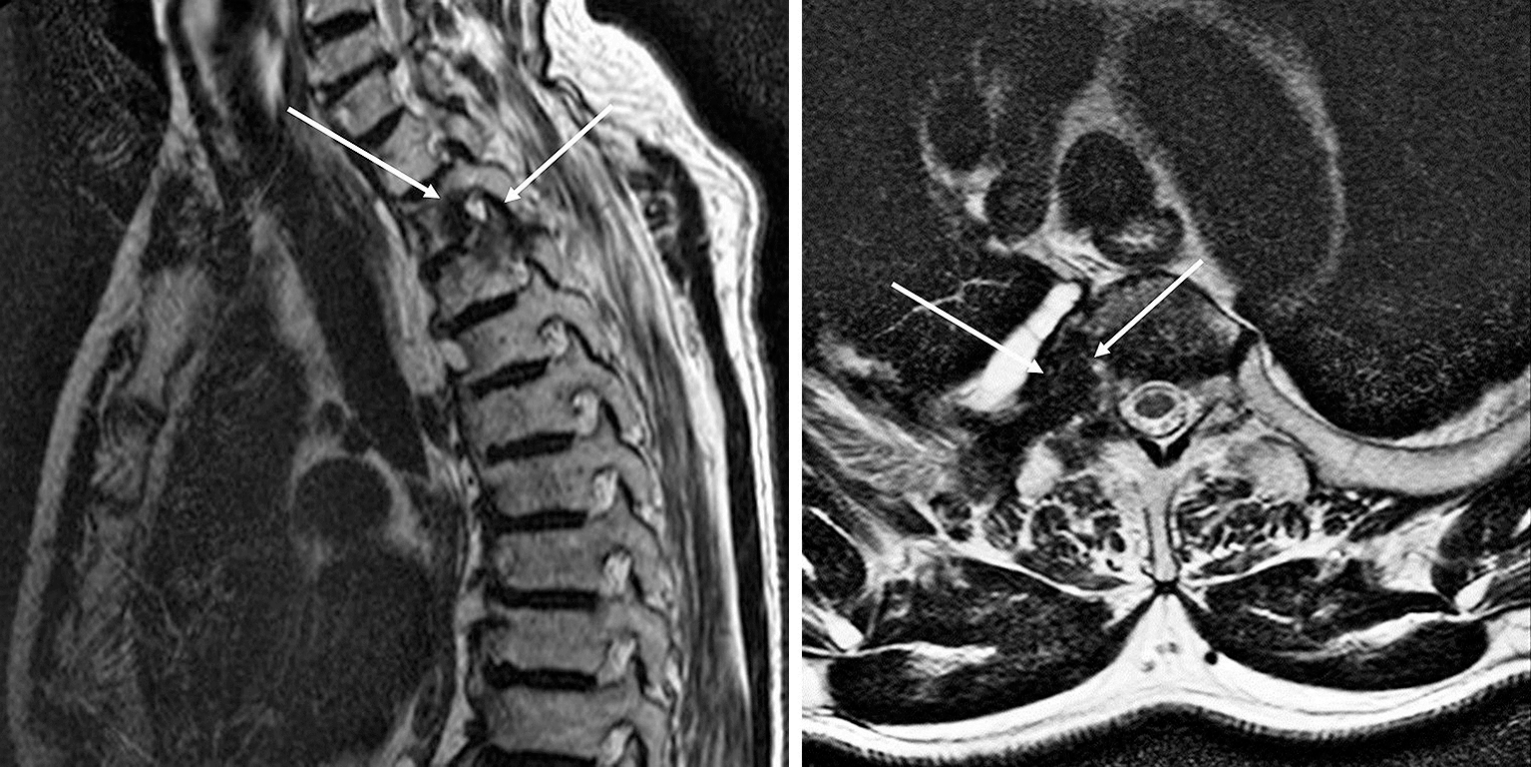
Magnetic resonance imaging of the thoracic spine showed tumor invasion in the intervertebral foramen.
